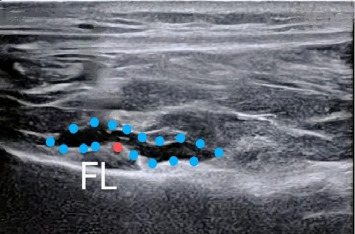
Ultrasound-guided superomedial genicular nerve block. Red circle: genicular artery, blue dotted line: spread of local anesthetic, and FL: femoral line.
